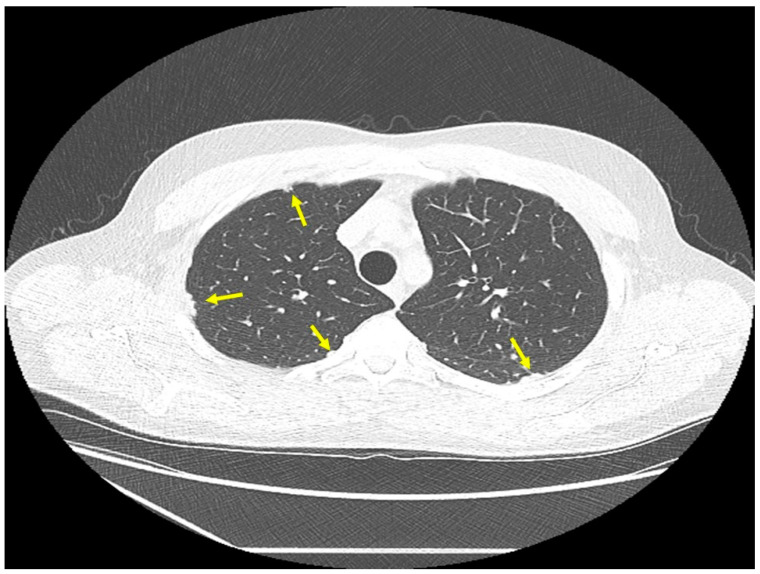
Chest CT image of a patient with pulmonary sarcoidosis, demonstrating subpleural opacities from granulomatous inflammation (arrows).
